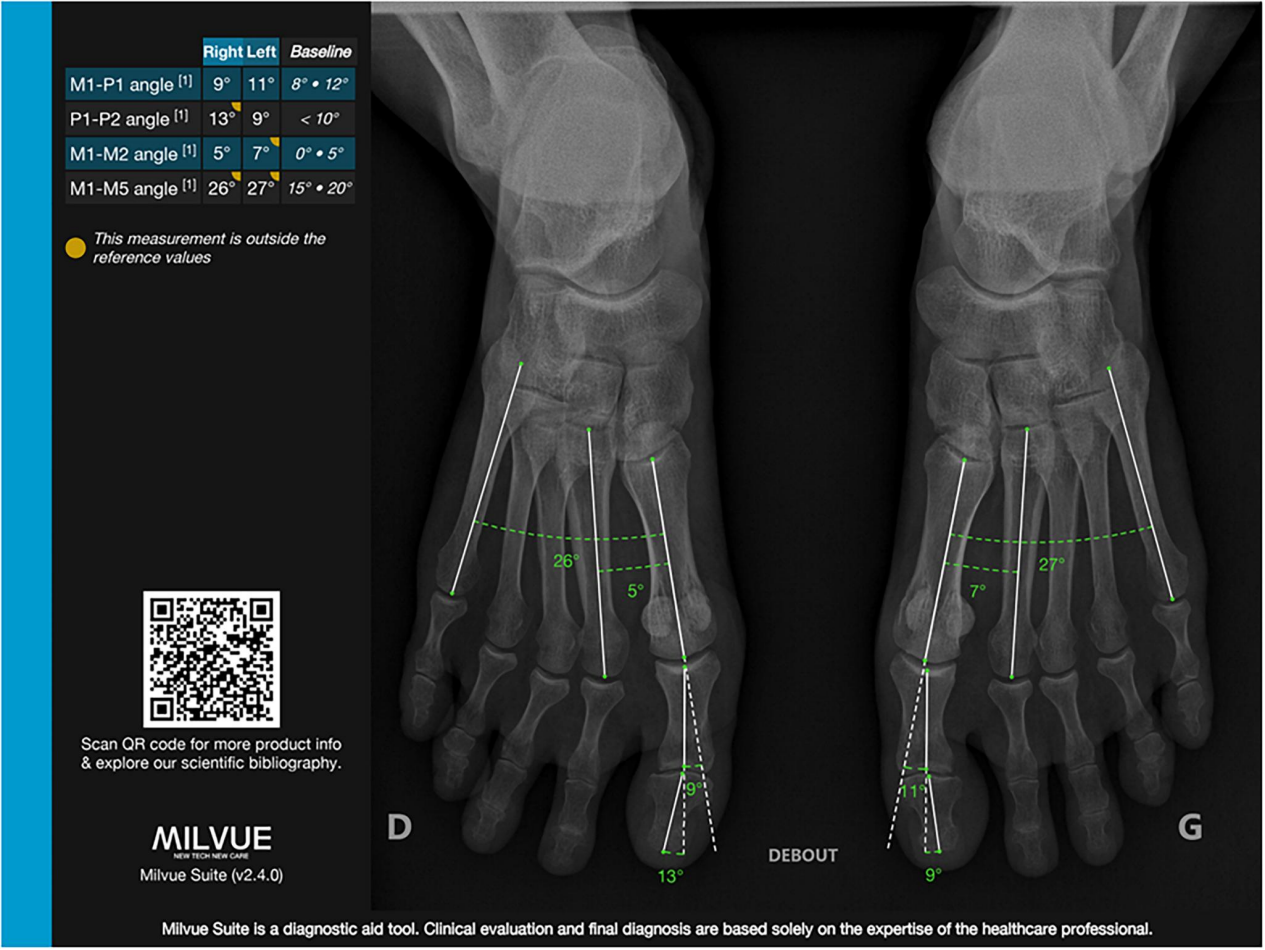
Automated measurements of feet radiographs, Milvue Suite (milvue).
